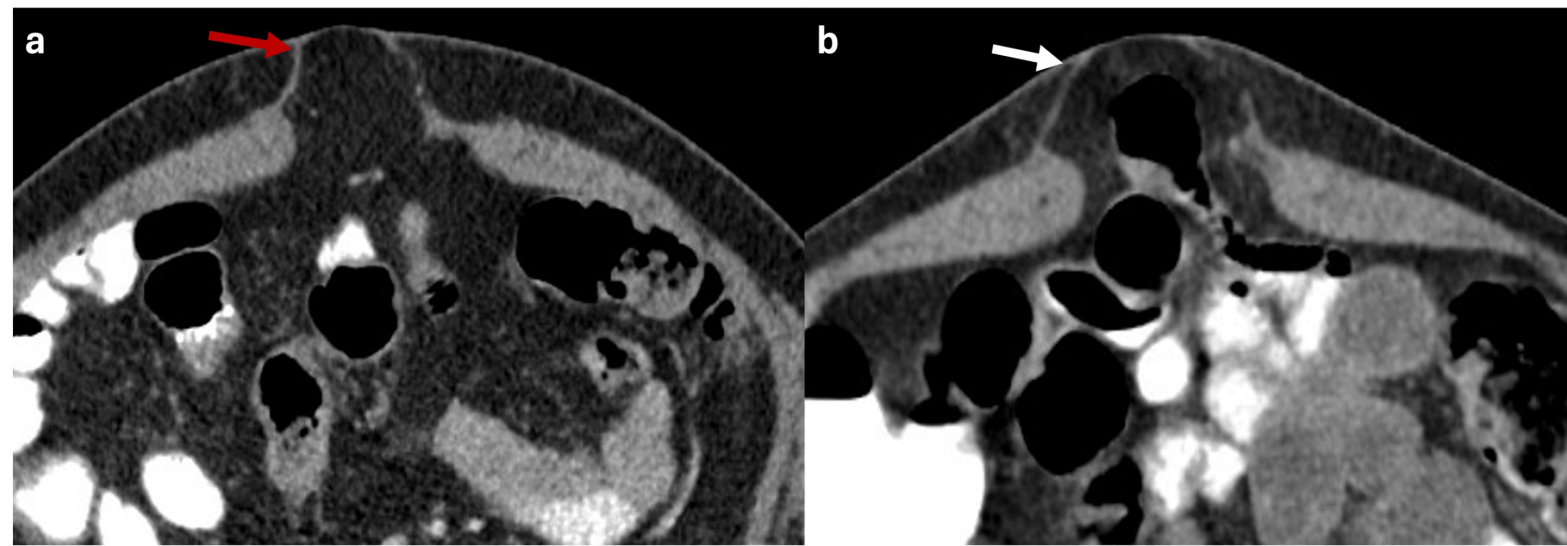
Axial CT scan of the abdomen with oral contrast showing how Valsalva maneuver modifies the content of the hernia sac (a, b) Axial CT scan of the abdomen with oral contrast showing a ventral abdominal wall hernia sac with fat content (red arrow). Image acquisition during the Valsalva maneuver increases the intra-abdominal pressure, showing that the hernia sac is then occupied by small bowel loops (white arrow). The figure was created by the authors.
